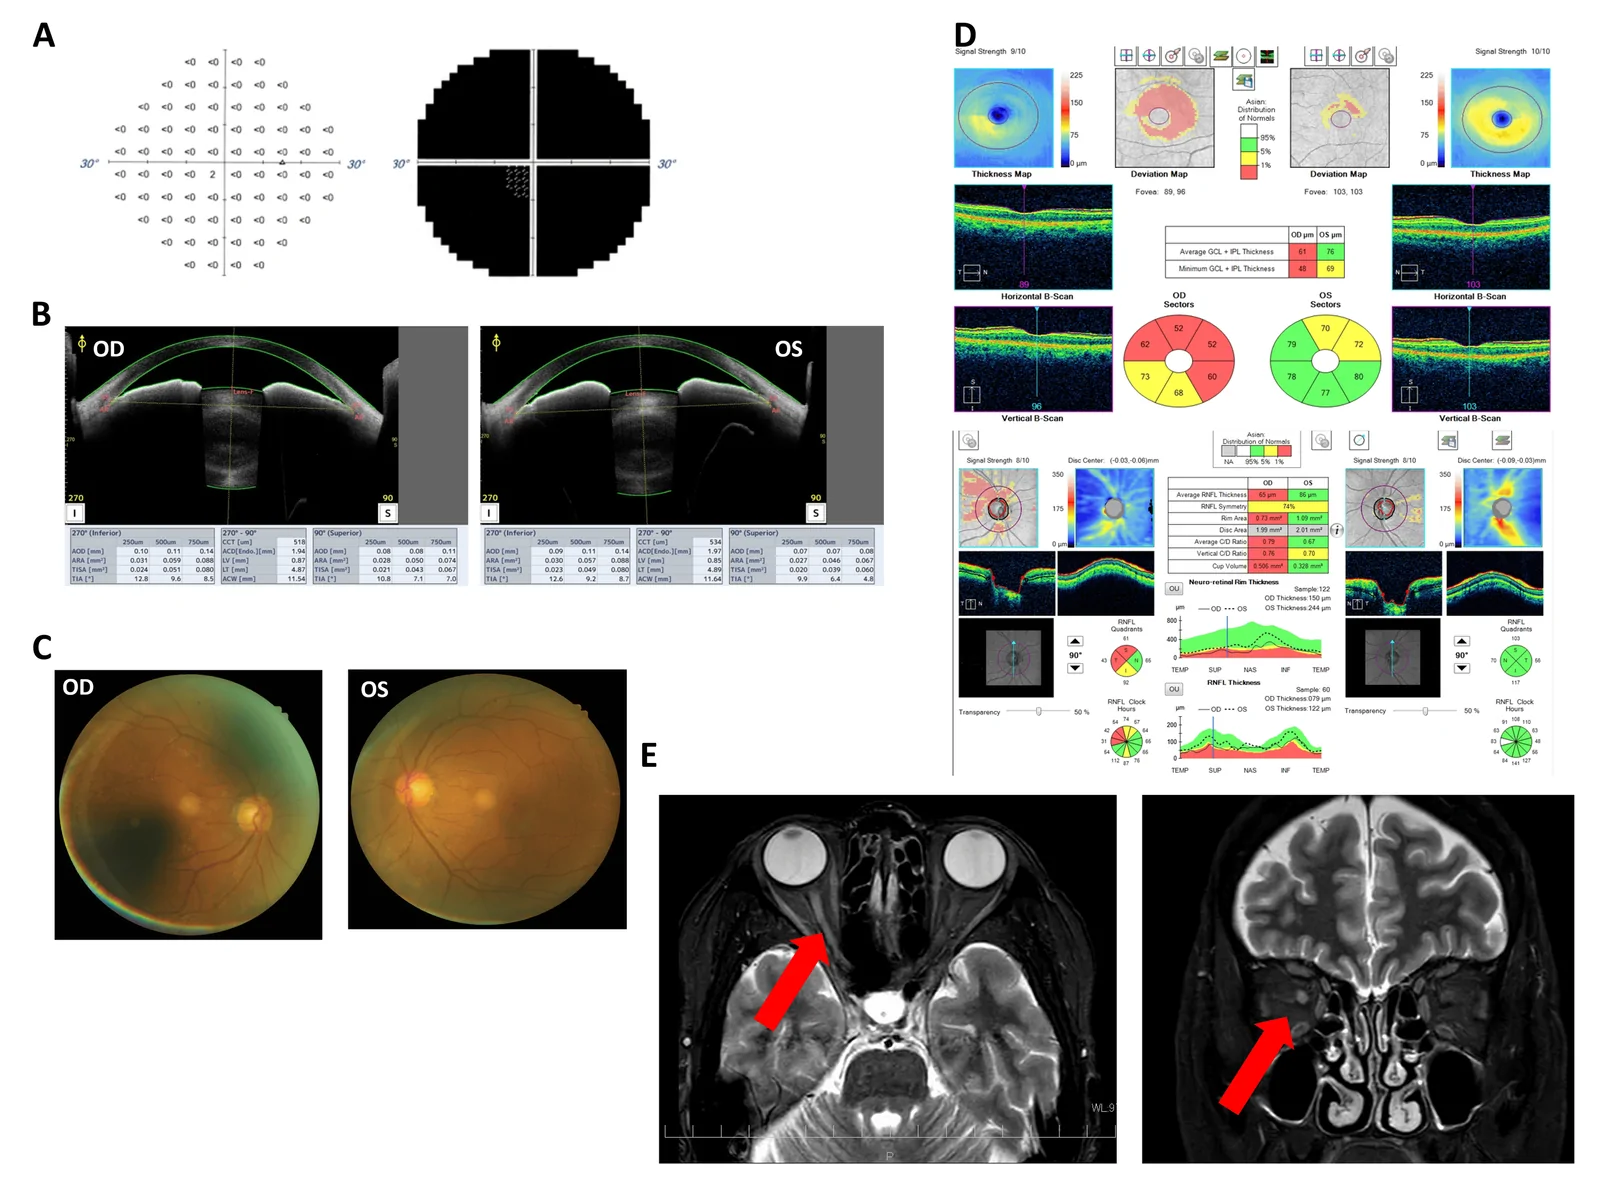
Ophthalmic and magnetic resonance imaging findings at presentation (A) Humphrey 30-2 visual field examination demonstrating profound visual field loss in the right eye. (B) Anterior segment optical coherence tomography demonstrating narrow anterior chamber angles in both eyes, supporting an angle-closure mechanism. (C) Color fundus photographs obtained without pharmacologic pupillary dilation because of concern for further IOP elevation in the presence of a markedly shallow anterior chamber. The apparent opacity over the macular/posterior pole region of the right eye represents an imaging artifact associated with the suboptimal image quality of nondilated fundus photography and does not represent a retinal lesion. (D) Macular ganglion cell-inner plexiform layer and circumpapillary retinal nerve fiber layer measurements obtained by optical coherence tomography. (E) Coronal and axial short tau inversion recovery magnetic resonance images demonstrating a hyperintense lesion in the anterior portion of the right optic nerve, supporting the diagnosis of optic neuritis. The arrow indicates the hyperintense lesion in the right optic nerve. OD, right eye; OS, left eye
